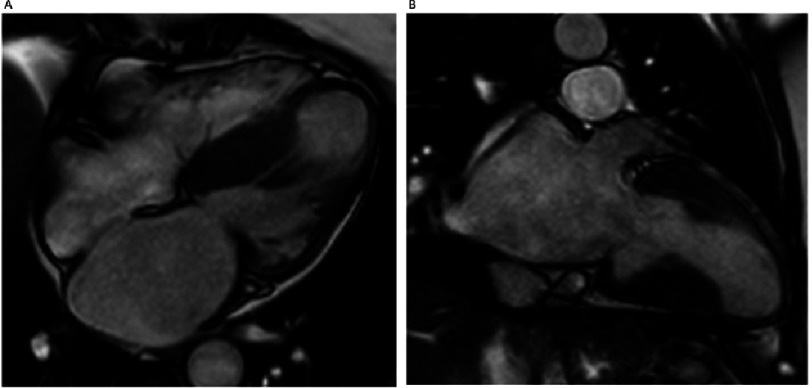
Cardiac Magnetic Resonance (CMR) cine apical 4 and 2 chamber view showing apical aneurism and mid-cavity hypertrophy. TNNI 3 p.Ala157Val.
